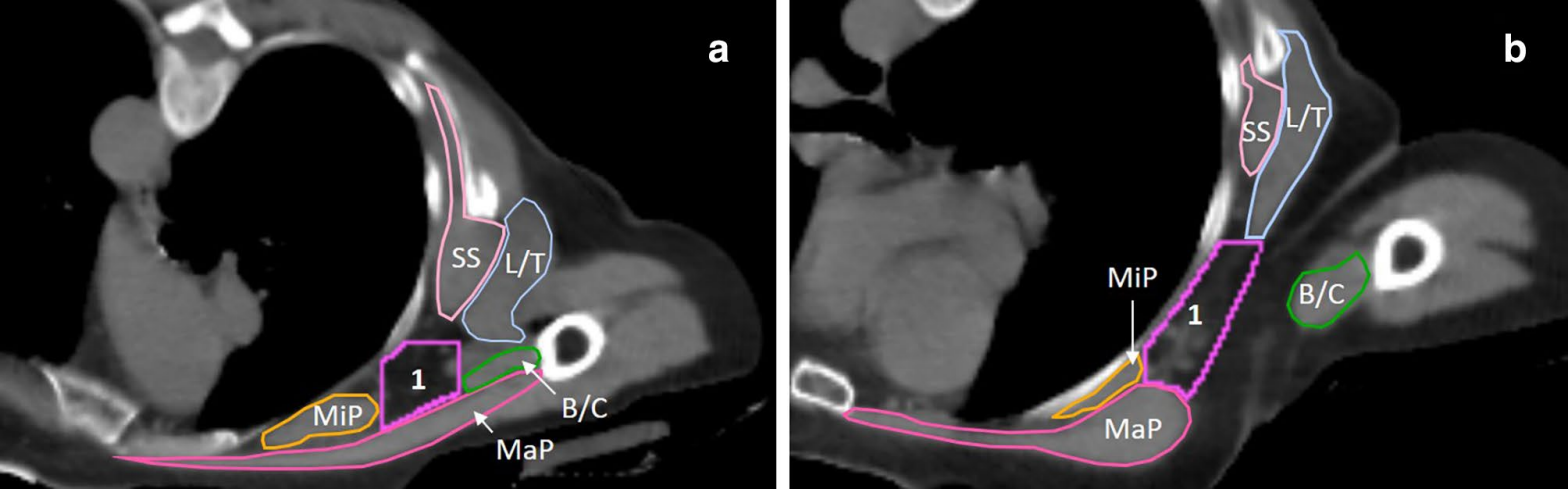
Adaptations to level I borders indicated on patient CT scan taken in PCP. (a) Shows a representation of the cranial slices, where the lateral border is defined by the medial edge of the biceps/coracobrachial muscle bundle, because the major pectoral muscle is still connected to the arm. Note that the dorsal border stops at the ventral edge of the latissimus dorsi/teres major muscle bundle here, because this reaches more ventrally than the ventral edge of the subscapular muscle at this level. (b) Shows a representation of the more caudal slices in level I  (of the same patient) where the major pectoral muscle is no longer connected to the arm and the lateral border is formed by connecting the lateral edge of the major  pectoral muscle to the ventral edge of the latissimus dorsi/teres major  muscle bundle. Light pink (SS) = subscapular muscle, light blue (L/T) = latissimus dorsi/teres major muscle bundle, purple (1) = level I, green (B/C) = biceps/coracobrachial muscle bundle, orange (MiP) = minor pectoral muscle, dark pink (MaP) = major pectoral muscle.
